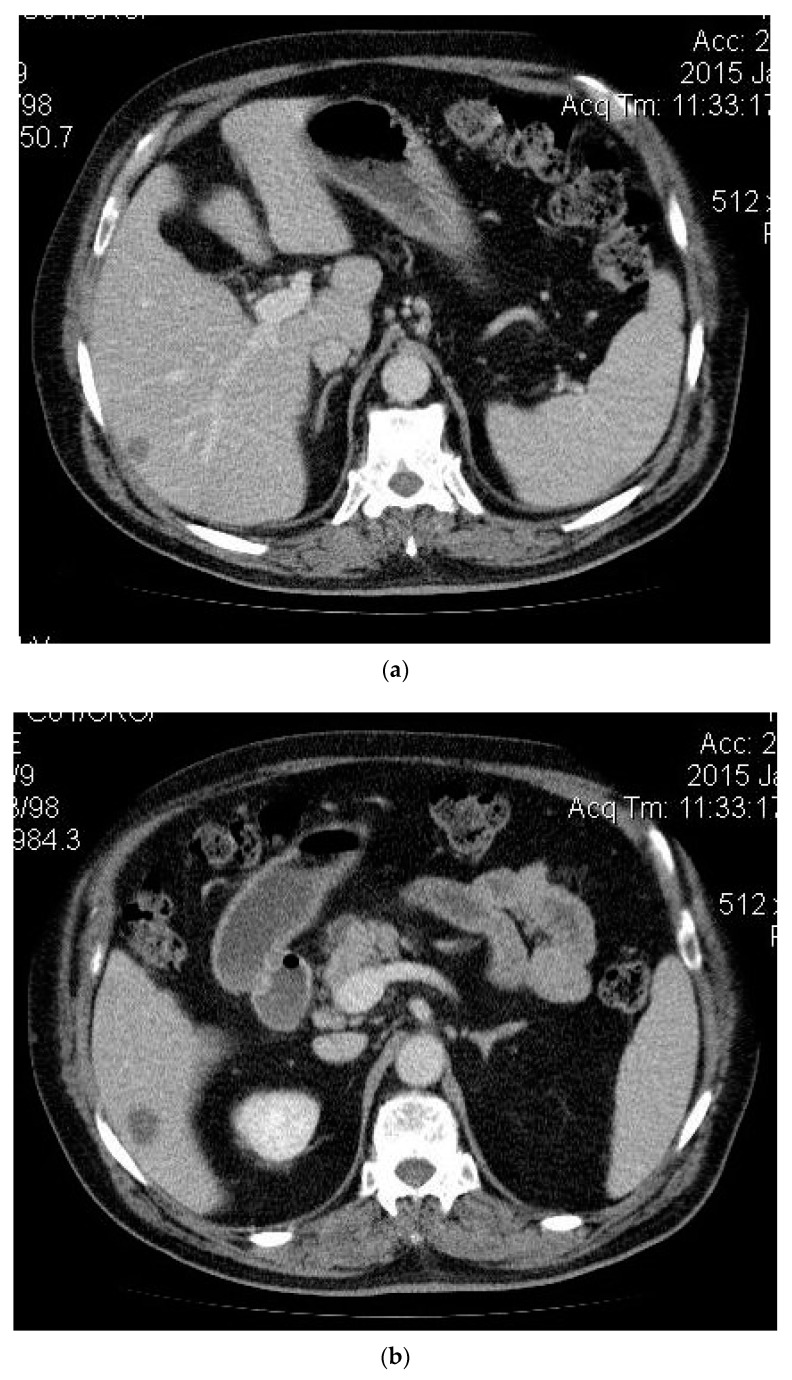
Preprocedure CT. (a) Metastatic lesion mass-1; (b) Metastatic lesion mass-2.
